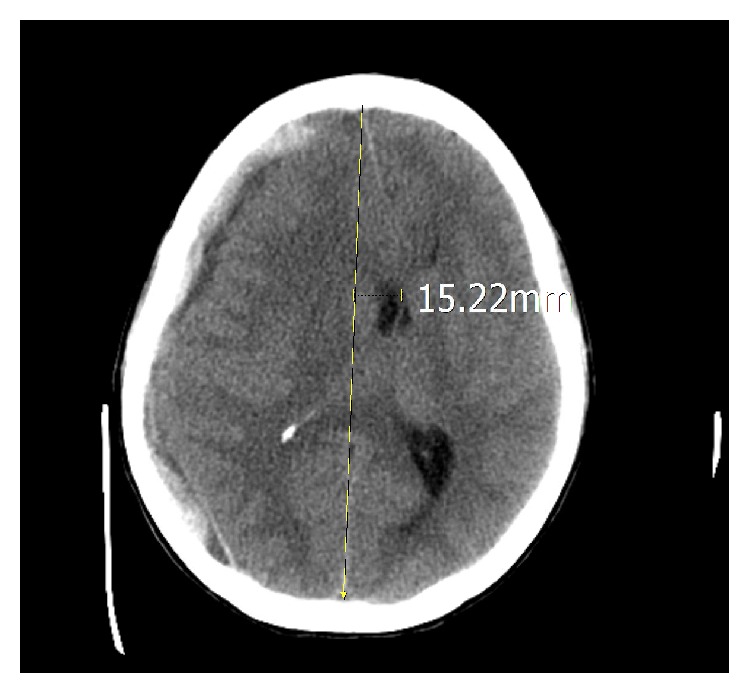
Computed tomography axial image showing right subdural hematoma with midline shift.
